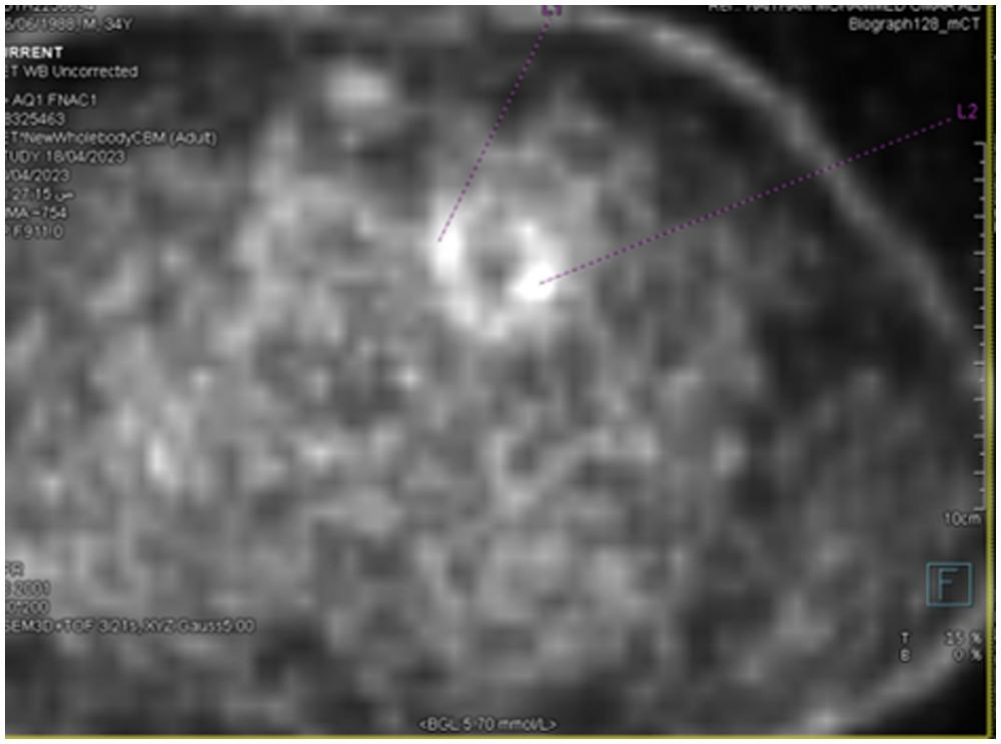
Axial non corrected FDG PET CT image.
